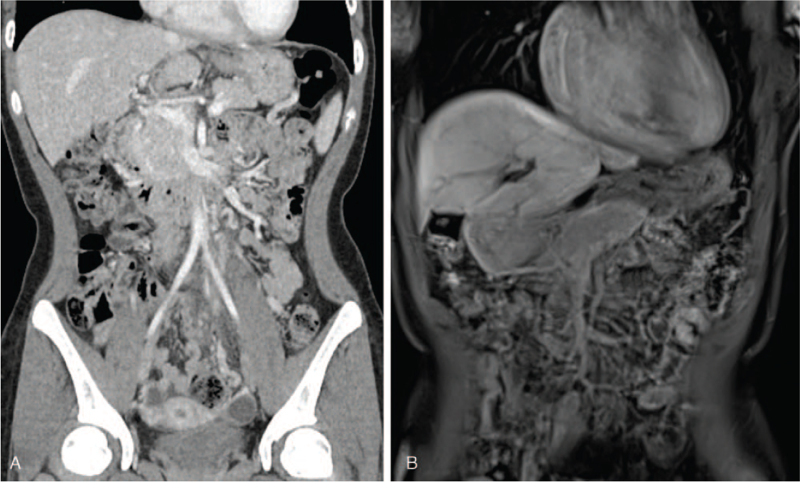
Computed tomography (CT) and magnetic resonance imaging after 9 months of warfarin treatment. (A) Diffuse thrombosis in inferior mesenteric vein (IMV) confluence is almost resolved on CT. (B) IMV thrombosis is improved. There is a contour change in the liver, and the hyperplastic lesion is slightly decreased.
